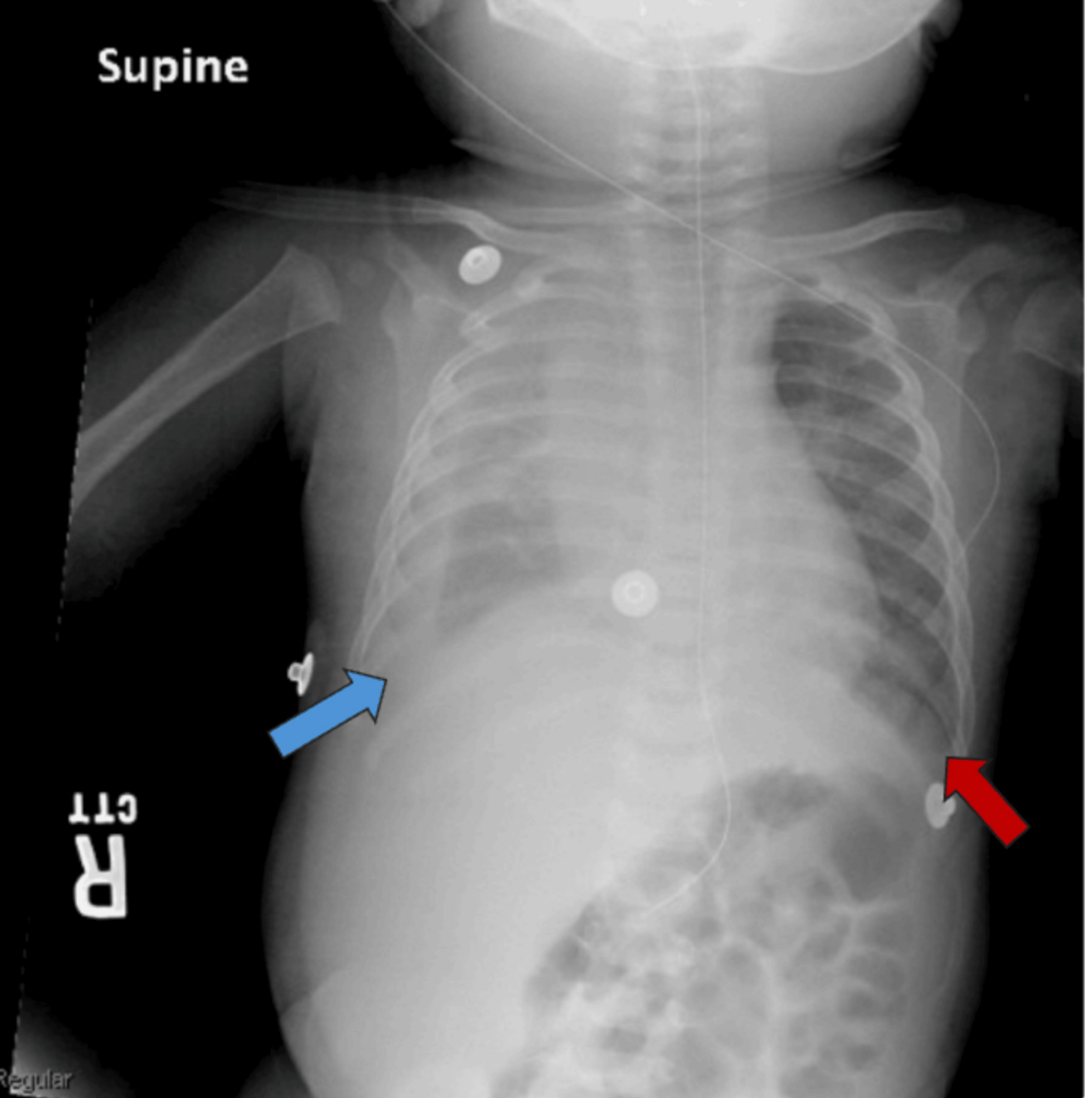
Frontal chest radiograph demonstrating a right-sided moderate pleural effusion (blue arrow) and a left-sided small-to-moderate pleural effusion (red arrow). No evidence of pneumothorax or acute osseous abnormalities.
